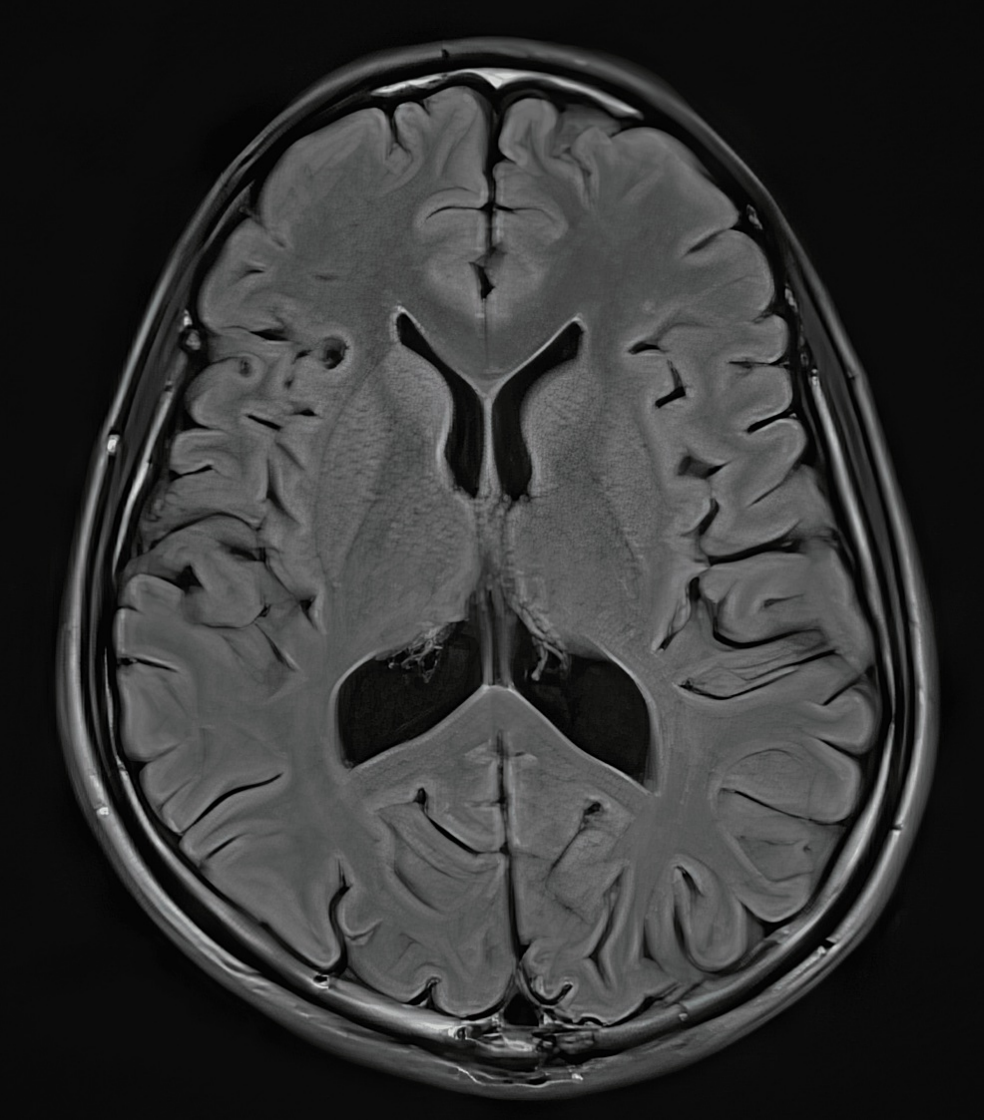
Normal MRI brain, FLAIR sequence FLAIR: Fluid-attenuated inversion recovery.
